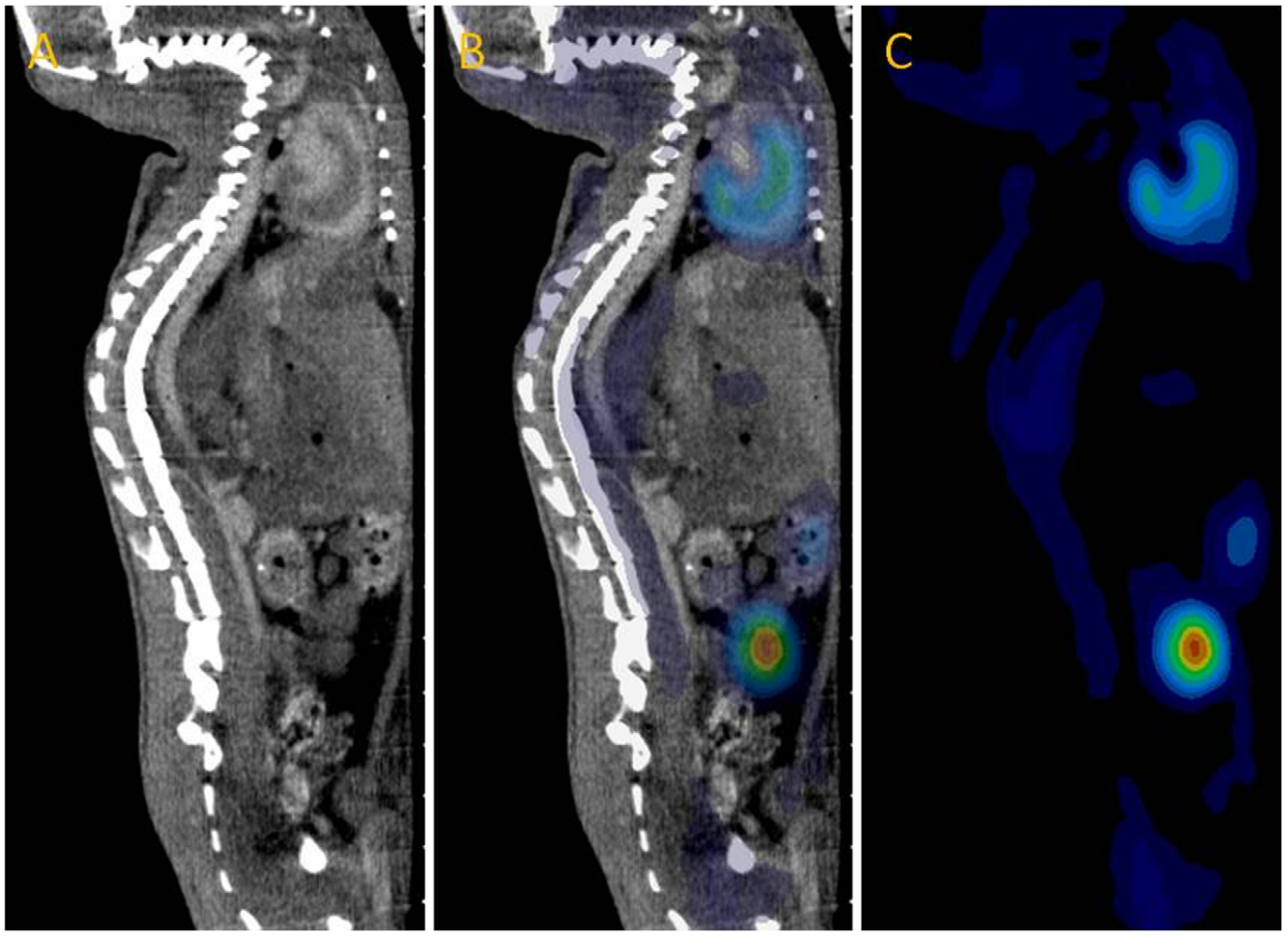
CT, fused PET/CT, and PET images. A Contrast-enhanced CT image. B Fused PET/CT image. C PET image. All images are in sagittal view.
